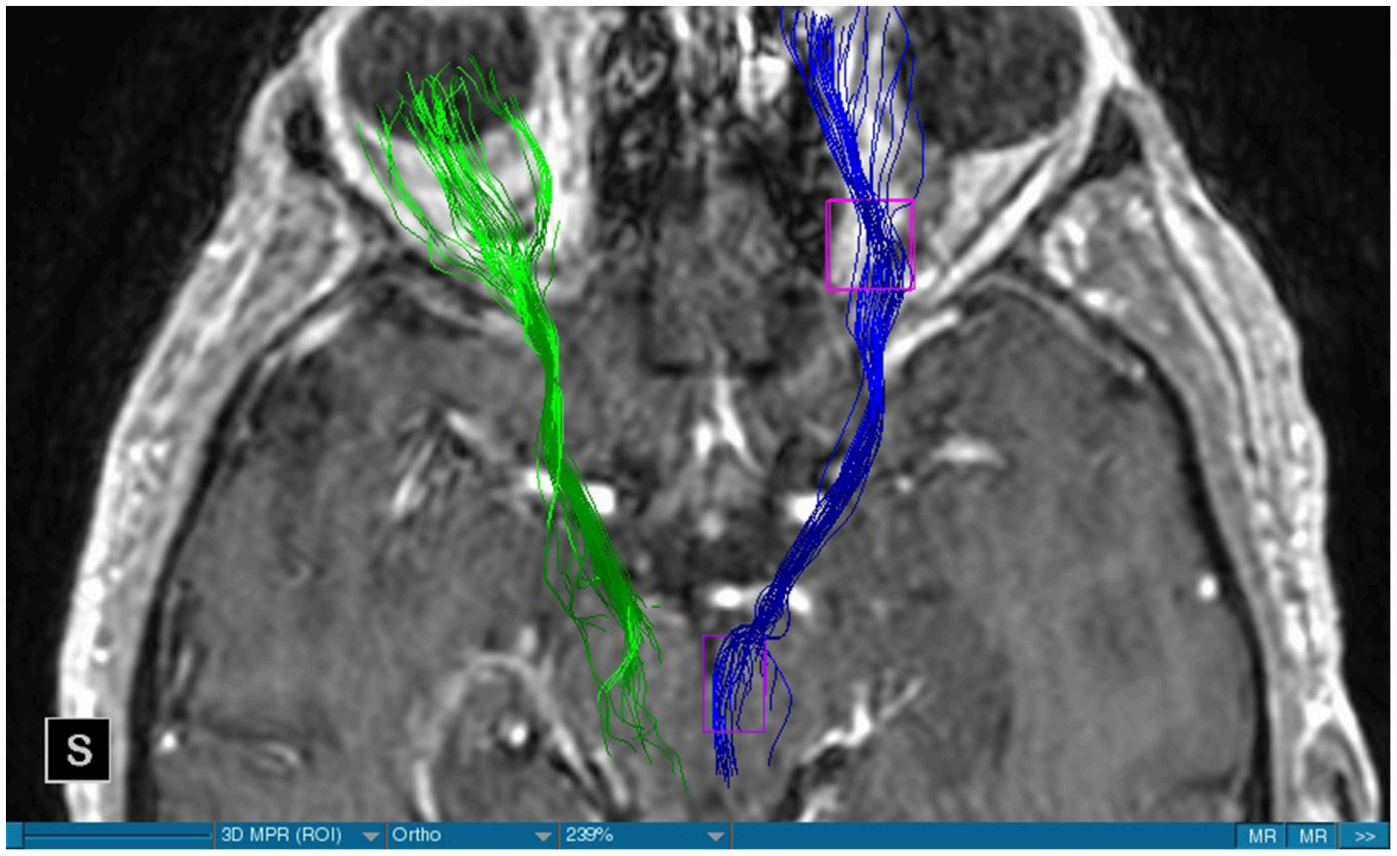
MRI axial view, T1, DBS, blue: right MFB, green: left MFB.
